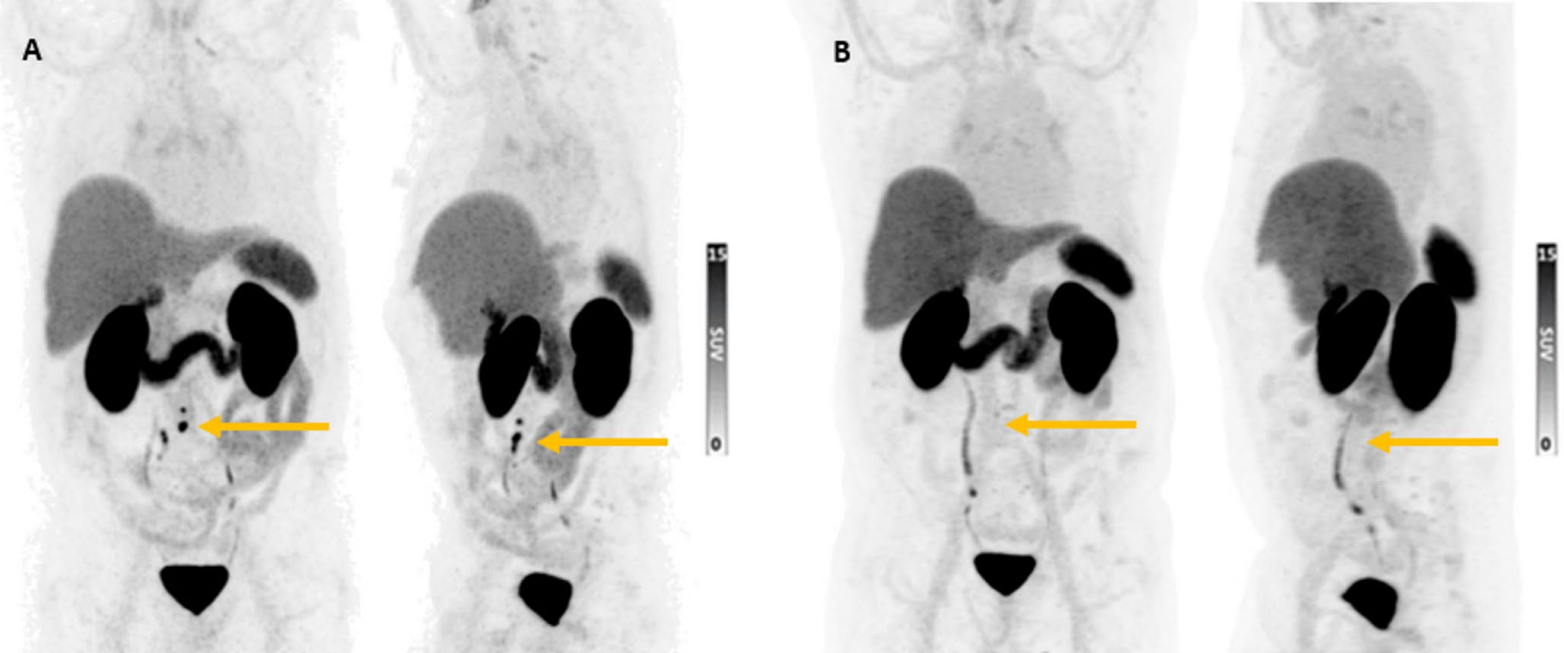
Therapy response with [^68^Ga]Ga-PSMA-11 PET images. [^68^Ga]Ga-PSMA-11 PET imaging of a patient (61 years) before (A) and after six RLT cycles of [¹⁷⁷Lu]Lu-PSMA-I&T (B). Yellows arrows in the baseline imaging indicate multiple PSMA-avid retroperitoneal lymph nodes metastases in the frontal and sagittal view. Yellow arrows in follow-up imaging show regression of PSMA-expressing lymph nodes, with no evidence of local recurrence or new osseous/visceral metastases. PSA declined from 2.54 ng/mL to 0.06 ng/mL (97.6% reduction). Over 13.9-month follow-up period, the patient showed no PSA progression and required no additional therapy
